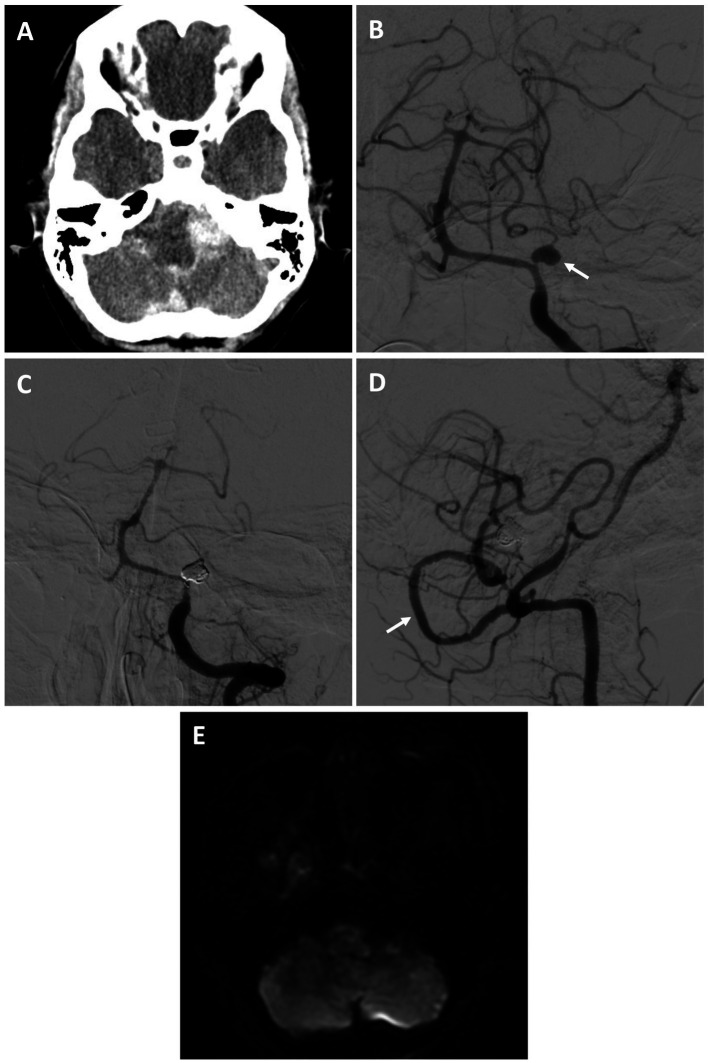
Patient #7. A 59-year-old female experienced a Hunt and Hess 3, Fisher 4 SAH from a left proximal PICA fusiform aneurysm [(A) axial non-contrast head CT; (B) AP angiogram of the left vertebral artery (arrow highlighting aneurysm)]. She underwent a V3 to PICA bypass using a DLCFA interposition graft, followed by internal PICA aneurysm embolization. She had no strokes and an uneventful recovery. On 4-month follow was performing all activities of daily living independently. Angiogram demonstrating PICA occlusion [(C) AP left vertebral artery injection, and patent bypass (D) lateral right vertebral artery injection (arrow highlights bypass)]. (E) Post-procedural MRI (axial DWI) demonstrated no strokes.
